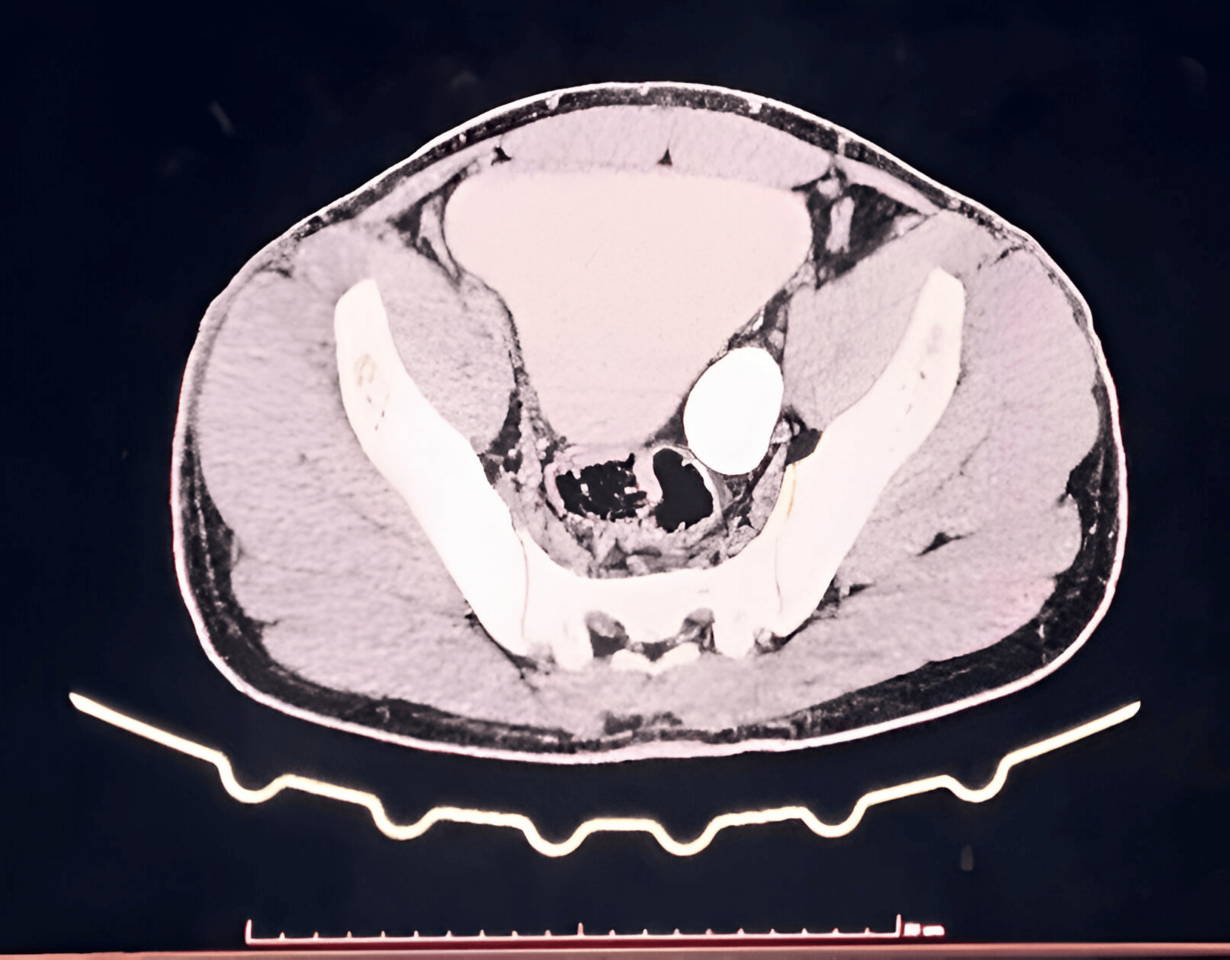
CT urogram showing left megaureter
